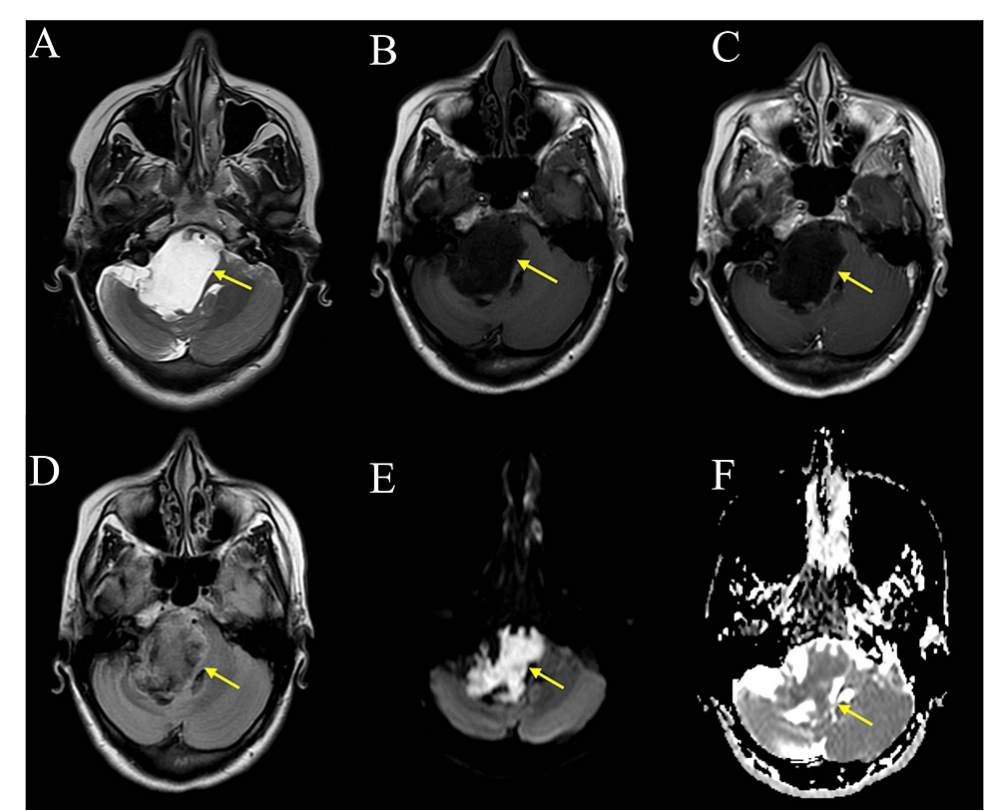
The MRI demonstrates a well-defined lobulated lesion centered in the right cerebellopontine angle cistern. The lesion is heterogeneous and follows cerebrospinal fluid signal intensity on T1 (A) (yellow arrow) and T2 (B) (yellow arrow) images, partially attenuated on FLAIR (C) (yellow arrow), and shows restricted diffusion on DWI (E, F) (yellow arrows). No enhancement is seen on the contrast-enhanced T1 (D) (yellow arrow). All the features indicate a diagnosis of an epidermoid cyst.
